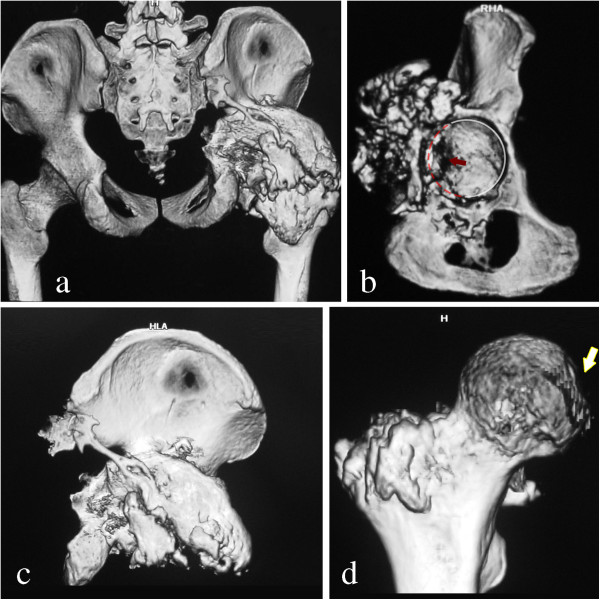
Three-dimensional CT reconstruction. (a) The massive osseous lesion in the posterolateral aspect of the acetabulum, partially connected with the posterior column, and surrounding the whole posterior hip joint from the roof of the acetabulum to the intertrochanteric line. (b,c) Bone defects and joint incongruence were revealed in the posterior wall (red dotted line and arrow in b). (d) Old impaction and degeneration exist at the posteroinferior portion of femoral head (white arrow in d). However, the weight-bearing portion of the femoral head had a normal appearance.
